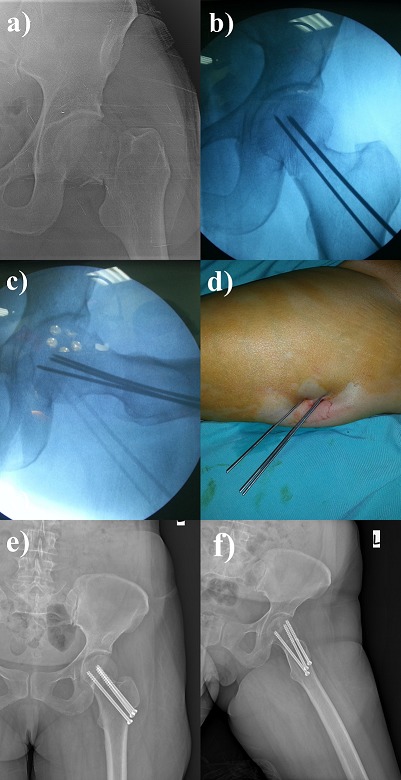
Radiographs of a 52 year-old woman with a left femoral neck fractures (Garden III fracture): Preoperative (a), intraoperative (b-c-d) and postoperative (e-f) radiographs
